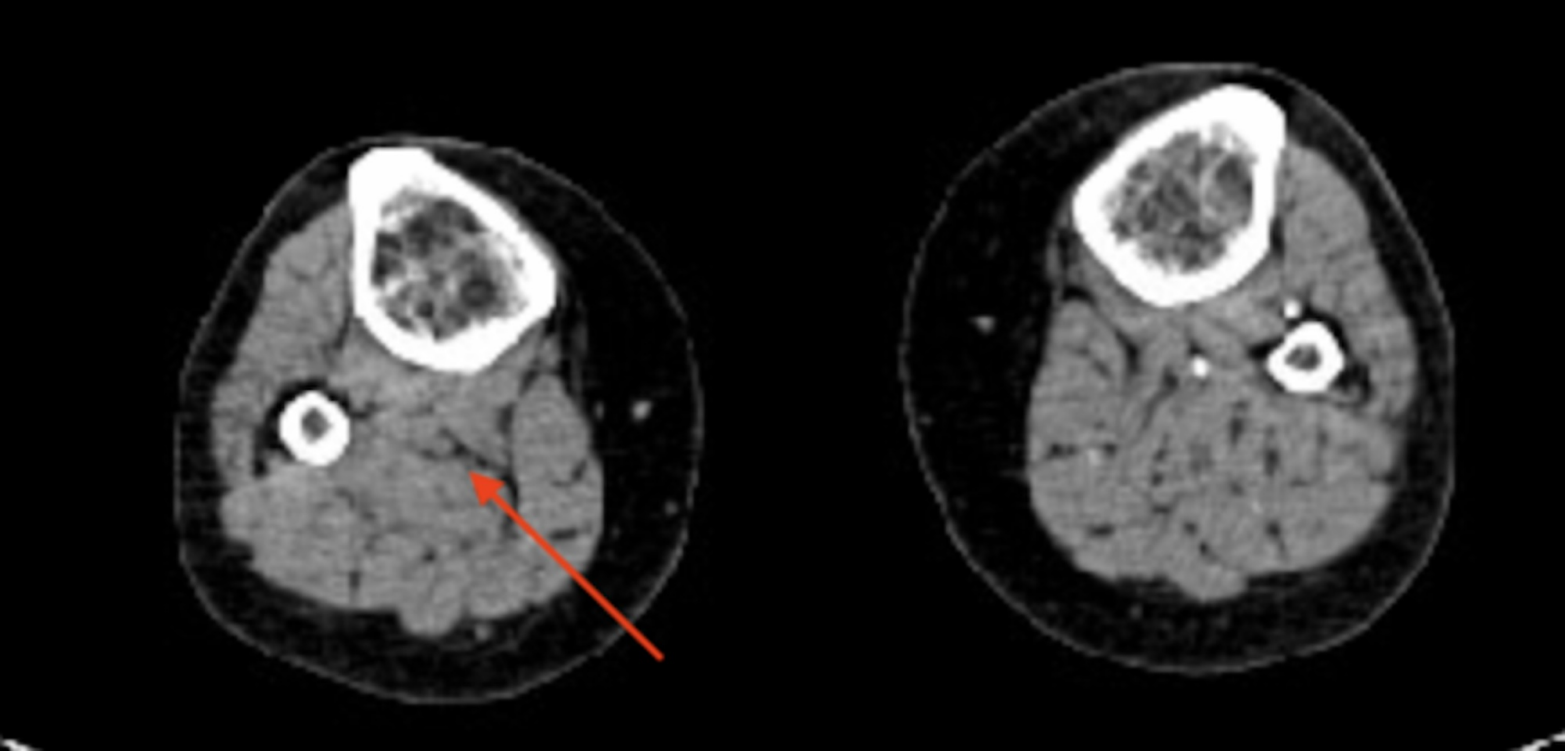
Computed tomography angiogram showing an occluded right popliteal artery (arrow)
